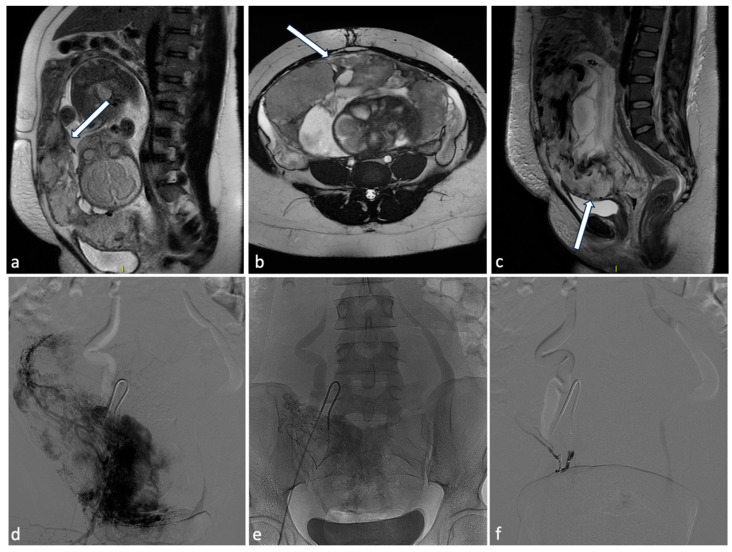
Placenta percreta. A 38-year-old woman at 27 weeks of gestation with placenta previa percreta. Sagittal (a) and axial (b) T2W SSFSE images show a heterogeneous placental signal with some low signal intensity bands representing venous lakes (arrows). There is also a focal uterine bulge with thinning of the overlying myometrium. The patient underwent a caesarean section and extraction of the fetus but no secondment due to placenta percreta. In the post-partum MRI (c), signs of placental percreta were still present with an image of bladder infiltration (arrow), later confirmed at cystoscopy. It was decided to perform endovascular devascularization of the placenta percreta left in situ before proceeding to hysterectomy. Preliminary arteriography of the abdominal aorta documented hypertrophy of both uterine arteries, clearly greater on the right, which exuberantly vascularized the hypertrophied placenta, supplying multiple intraplacental high-flow vascular lakes (d). After superselective catheterization with a coaxial microcatheter of both uterine arteries and some of their distal branches, bilateral embolization was performed by infusion of a suspension of embolizing particles. The procedure was completed with release of 3 platinum microspirals (3 mm diameter) at the level of the distal right uterine artery (e), proximal to the origin of the main collaterals and 2 microspirals (3 mm) at the origin of the left uterine artery. At the end of the operation, satisfactory uterine and placental devascularization was shown (f).
